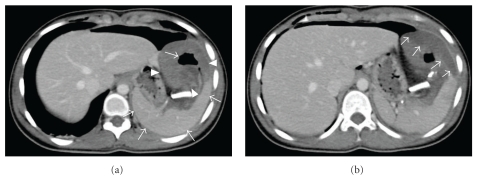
(a, b) Axial enhanced CT postsurgical decapsulation of the splenic cyst (3rd post-operative day) reveals an intact and homogeneously enhanced splenic parenchyma (a). The hypodense area at the anatomic region of the decapsulated cyst anterior to the spleen (a) that contains loculation of air in its center (a) represents the remnants of the cystic wall that have partially collapsed after laparoscopic surgical decapsulation together with perisplenic pockets of fluid (b).
